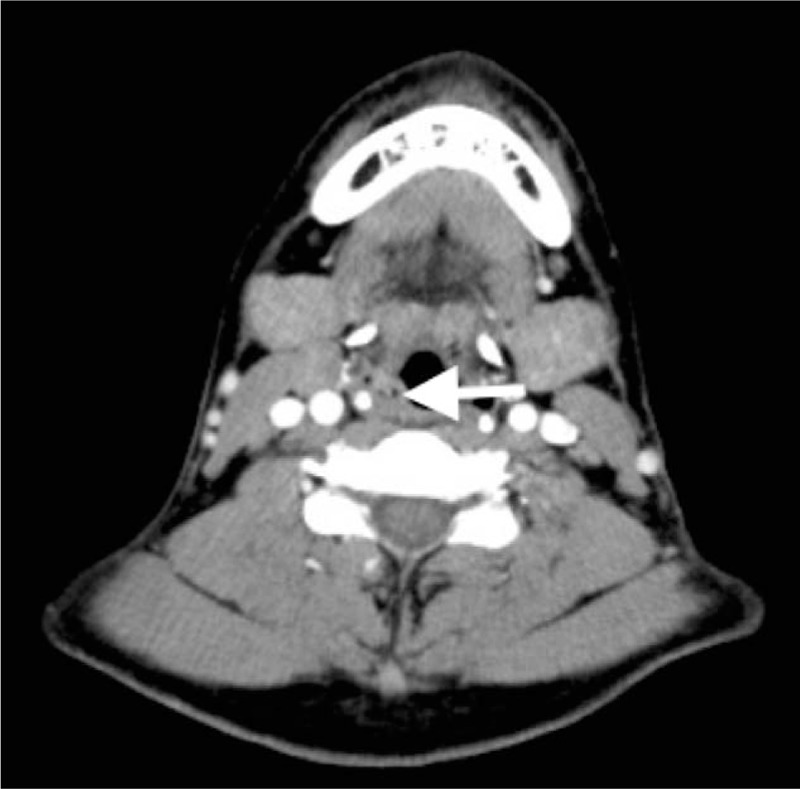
CT scan showing a hypodense mass in larynx (arrowhead).
